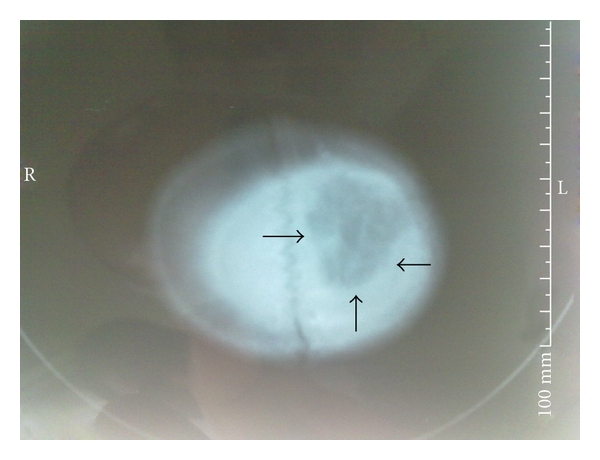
Axial computed tomography scan of the head (taken at time of presentation) through the left frontoparietal region shows a large subgaleal abscess.
